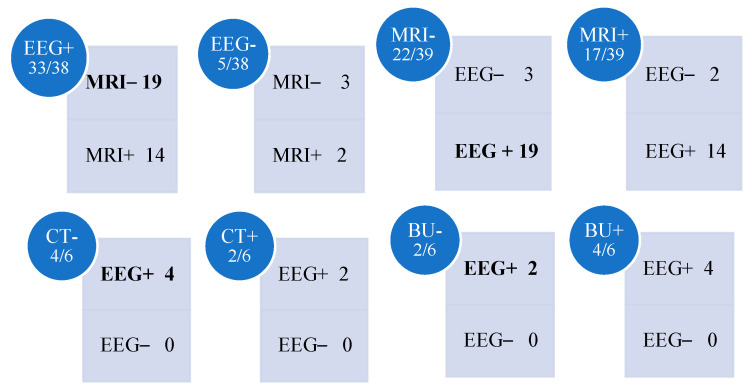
Matrices showing the relationships between the different diagnostic methods. Legend: BU: brain ultrasound. CT: brain computed tomography; EEG: electroencephalography MRI: brain magnetic resonance imaging.
